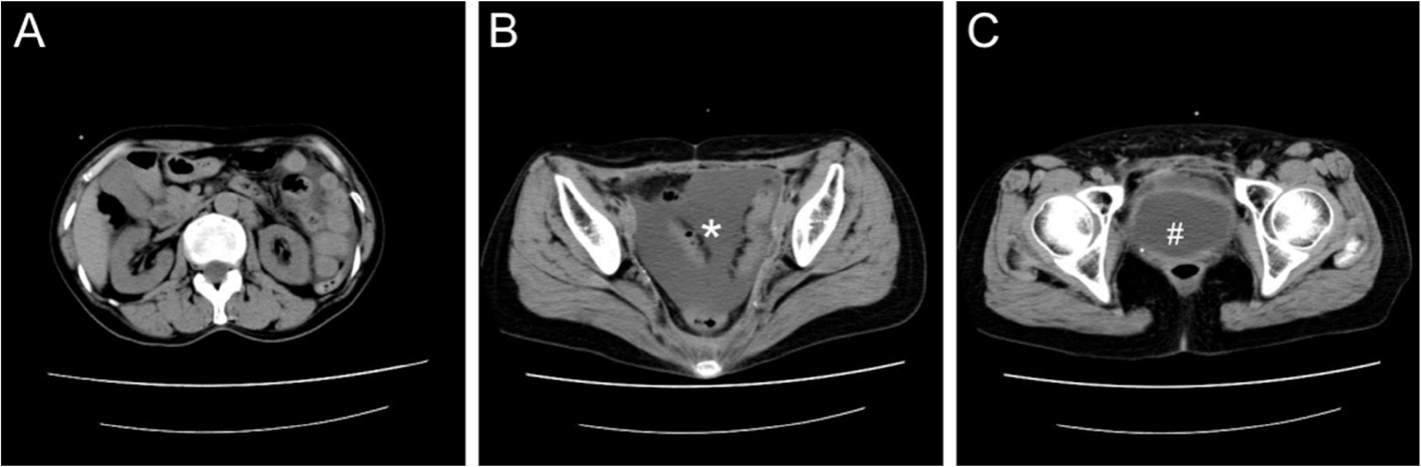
Unenhanced computed tomography images. (A) At the kidney level, the kidneys were normal size and hydronephrosis was not evident. (B) At the pelvic level, intraperitoneal fluid collection (*) was observed. (C) At the urinary bladder (#) level, neither blood attenuation nor bladder wall abnormalities were identified.
